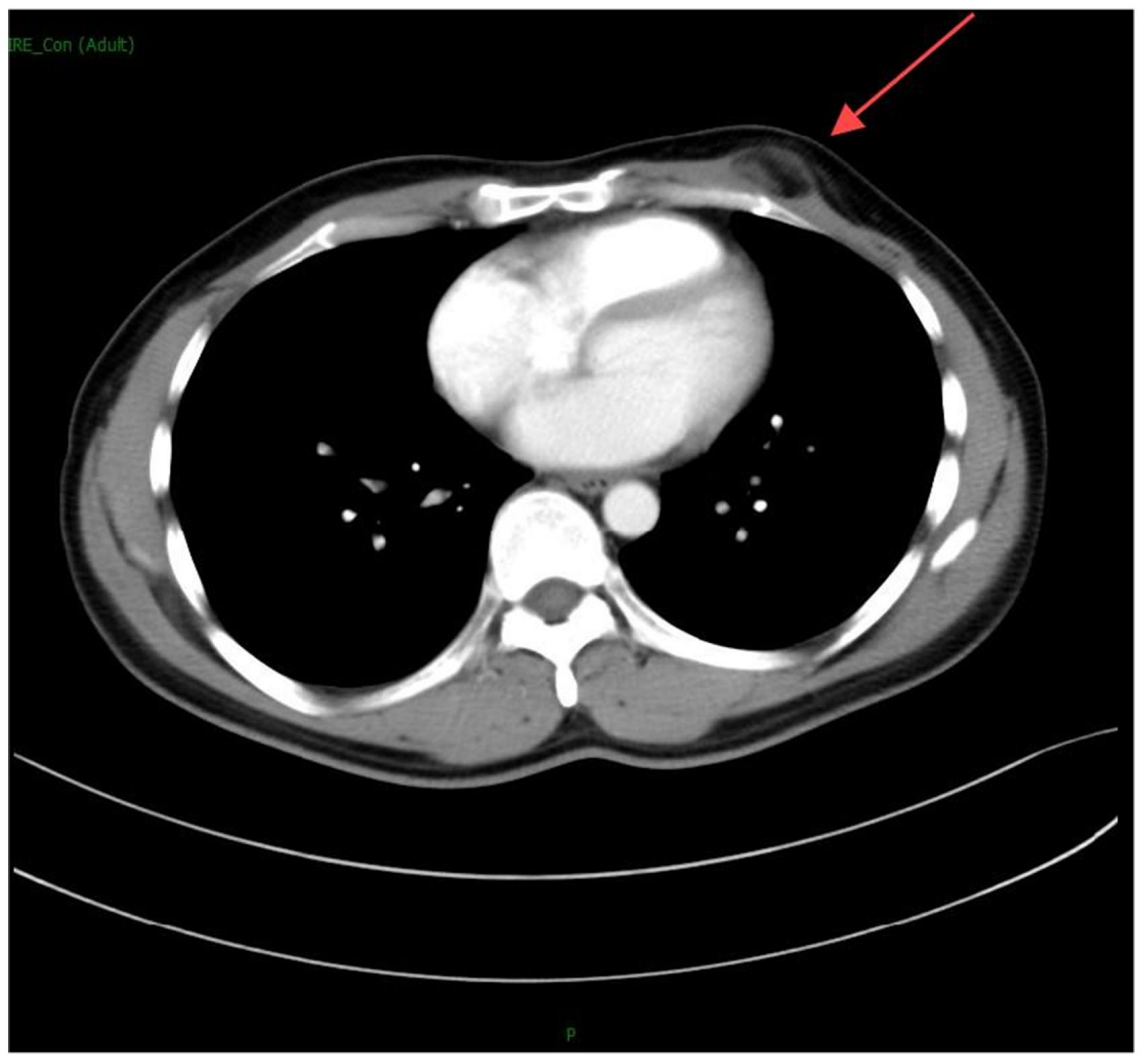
Contrast-enhanced chest CT scan showing no significant enhancement of the left chest wall nodule.
